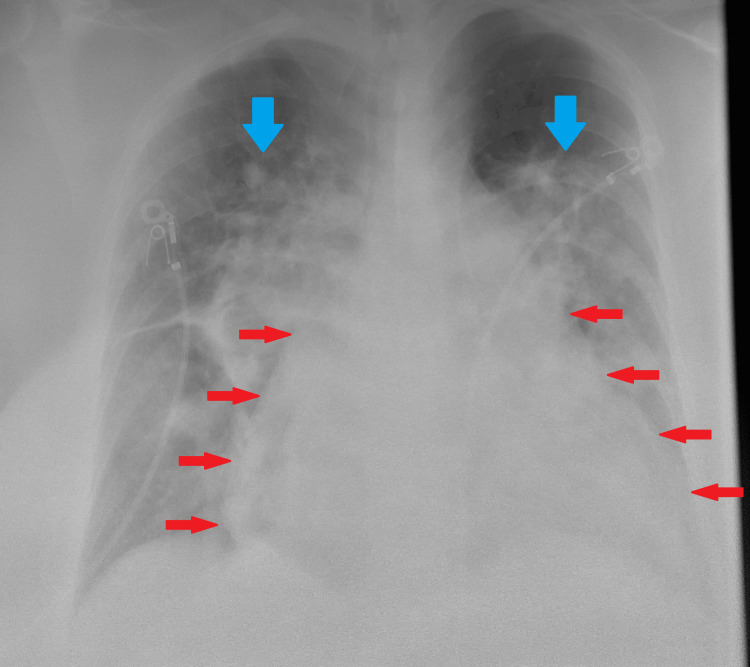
Anterior-posterior chest x-ray The chest radiograph in the anterior-posterior view of the patient reveals an enlarged cardiomediastinal silhouette (cardiac silhouette outlined by red arrows) and notable diffuse bilateral pulmonary opacities with a perihilar distribution (blue arrows).
